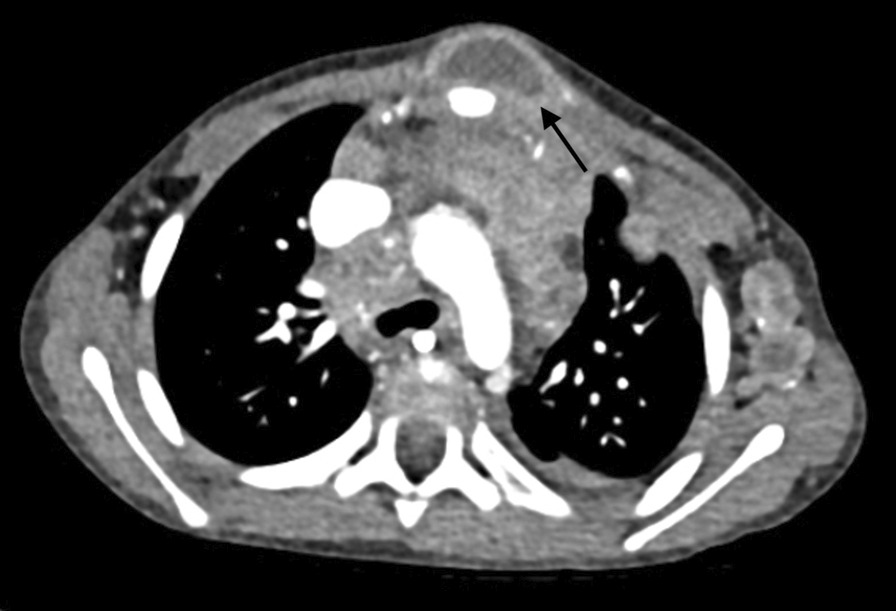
Computed tomography image demonstrating the presternal low-density mass with rim enhancement suggestive of an abscess (shown by arrow)
